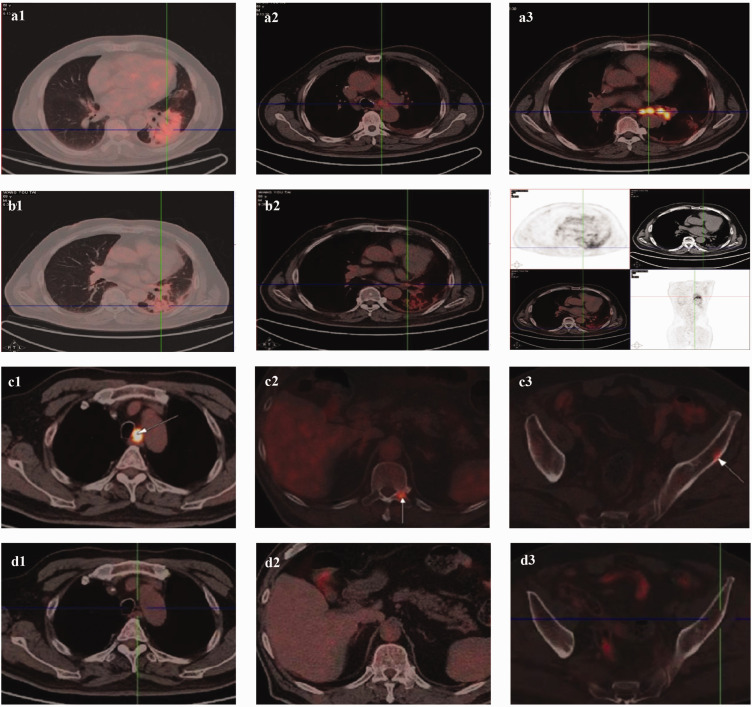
(a) Baseline chest PET/CT scan in August 2019. (a1) Lesion in the lower lobe. (a2) Lesion in the mediastinum. (a3) Lesion in the left hilum and left main bronchi. (b) PET/CT scan showing a new shadow in the lower lobe of the left lung in May 2020. (b1) Lesion in the lung window. (b2) Lesion in the mediastinal window. (b3) Lesion in all windows. (c) PET/CT in March 2021 showing (c1) enlarged lymph nodes in the left posterior trachea of the mediastinum, as well as new lesions in the (c2) left pedicle of the 11th and 12th thoracic vertebrae and (c3) left iliac wing. (d) PET/CT in April 2021 showing the lesions after two cycles of paclitaxel (albumin-bound) and carboplatin combined with the PD-L1 inhibitor durvalumab. Lesions in the (d1) mediastinum, (d2) left pedicle of the 11th and 12th thoracic vertebrae, and (d3) left iliac wing are compared with those from March 2021 and (e) PET/CT in October 2021 showed new lesions in the (e1) mediastinum, (e2) hilum, and (e3) lower lobe of the left lung.
